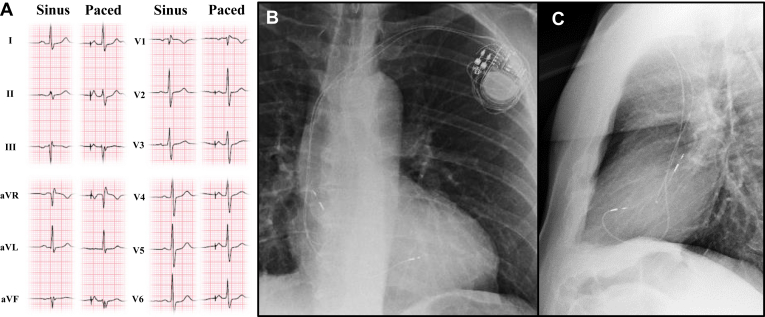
P-wave characteristics of Bachmann bundle pacing. A: Sinus rhythm and high right atrial paced rhythm from a patient in the high right atrial septal pacing cohort who met all criteria for Bachmann bundle pacing. Note the tall and narrow P waves best appreciated in the inferior leads. B, C: Chest radiographs in posteroanterior (B) and lateral (C) views showing atrial lead position in the same patient and the ventricular lead in the typical position for left bundle branch area pacing.
